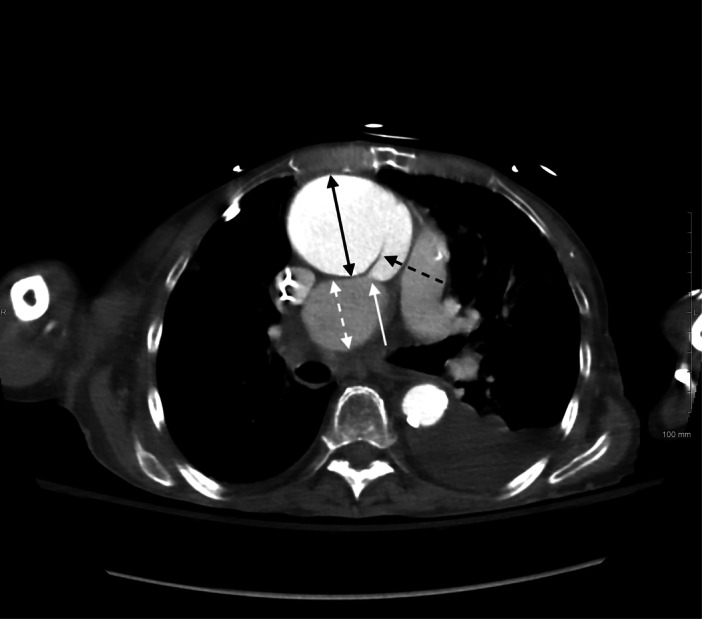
CT angiography of the aorta. There is an aneurysmal dilation of the ascending thoracic aorta measuring up to 6.1 cm in diameter (black double‐sided arrow) with a focal dissection flap (black dashed arrow) in the distal ascending thoracic aorta extending into the arch. There is a focal mass in the mediastinum measuring 3.1 × 4.3 cm (white dashed double‐sided arrow) demonstrating enhancement on postcontrast images that likely represents pseudoaneurysm. Communication between the false lumen of the aortic dissection and focal mediastinal mass is depicted with the white solid arrow.
